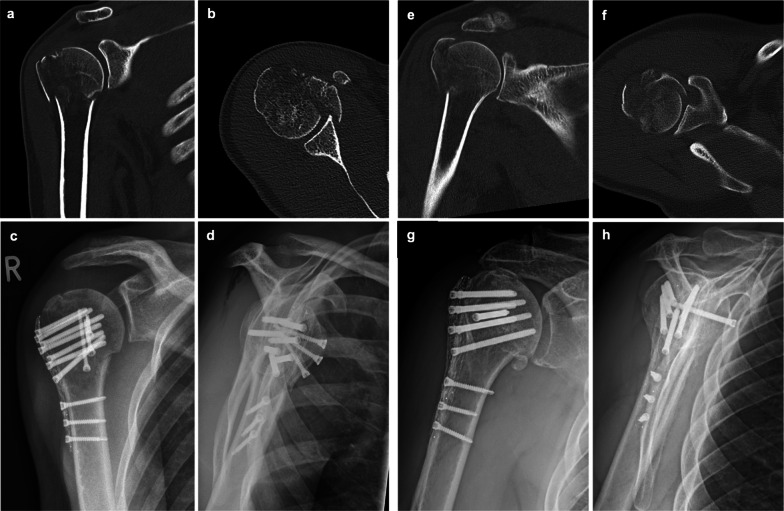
51-year-old female patient with four-part proximal humerus fracture and head split component treated with a CFR-PEEK plate osteosynthesis and additional screws for the lesser tuberosity: a preoperative CT scan in a coronal plane; b preoperative CT scan in an axial plane; c postoperative radiograph in AP view; d postoperative radiograph in lateral view; 58-year-old male patient with four-part proximal humerus fracture treated with a CFR-PEEK plate osteosynthesis and an additional screw for the lesser tuberosity; e preoperative CT scan in a coronal plane, f preoperative CT scan in an axial plane; g postoperative radiograph in AP view; h postoperative radiograph in lateral view. *CFR-PEEK* carbon-fibre-reinforced polyetheretherketone, *CT* computed tomography
